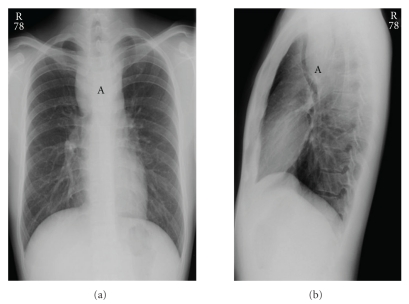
Chest X-ray AP (a) and lateral (b) views showing a large mediastinal mass (A).
